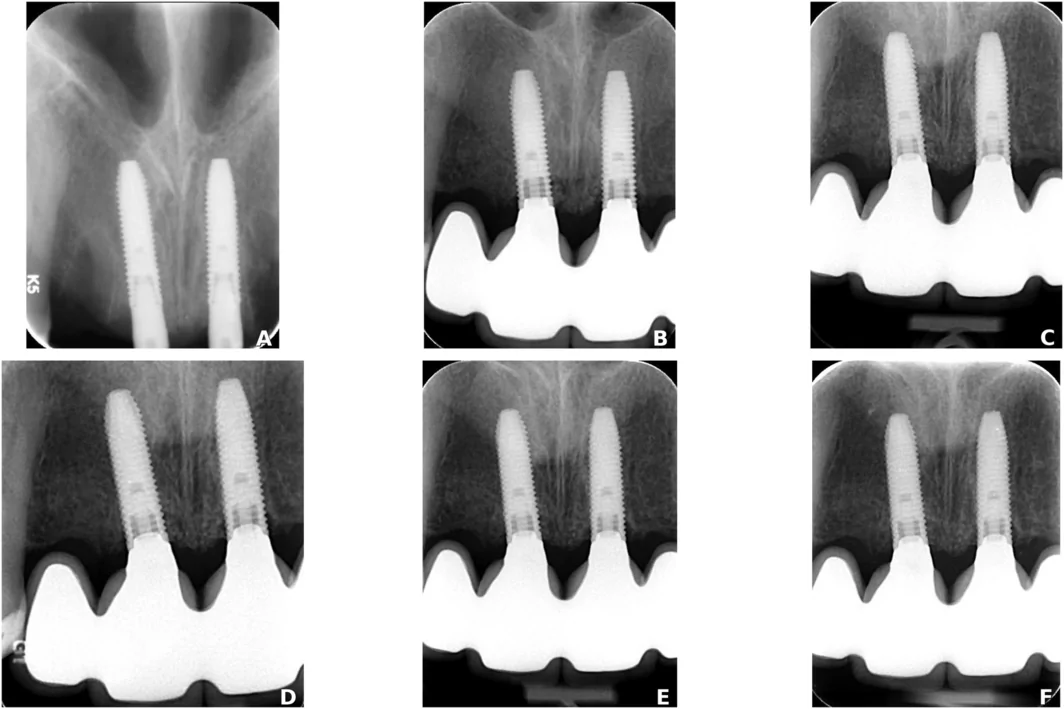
Serial periapical radiographs documenting implant positioning, osseointegration, and marginal bone levels over a 6‐year follow‐up period. (A) Post‐surgical periapical radiograph obtained immediately after implant placement, demonstrating implant positioning and surrounding bone adaptation, obtained on January 25, 2019. (B) One‐year follow‐up periapical radiograph showing satisfactory osseointegration and stable marginal bone levels, obtained on December 16, 2020. (C) Three‐year follow‐up periapical radiograph showing satisfactory osseointegration and stable marginal bone levels, obtained on April 25, 2022. (D) Four‐year follow‐up periapical radiograph showing satisfactory osseointegration and stable marginal bone levels, obtained on January 30, 2023. (E) Five‐year follow‐up periapical radiograph showing satisfactory osseointegration and stable marginal bone levels, obtained on January 16, 2024. (F) Six‐year follow‐up periapical radiograph showing satisfactory osseointegration and stable marginal bone levels, obtained on February 24, 2025.
